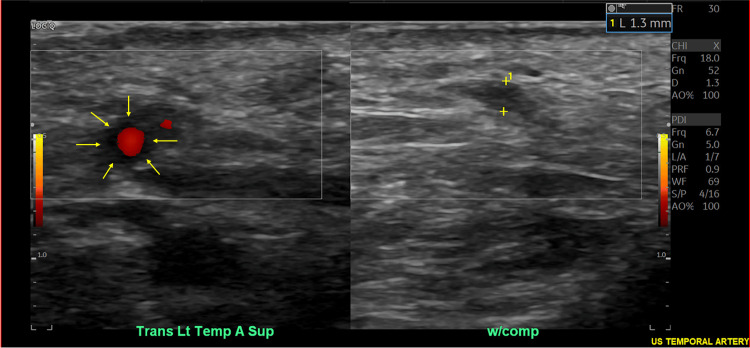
Temporal artery ultrasound revealing "halo sign" of the left temporal artery (left frame, arrows).
